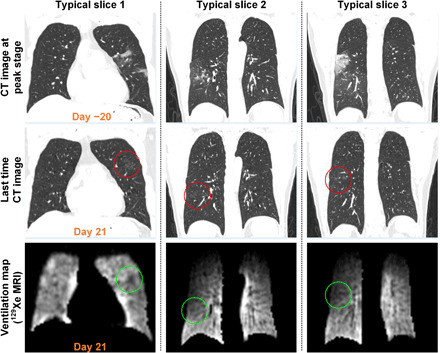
Lung images for subject 2. Serial chest CT images (top row: day −20, three slices; second row: day 21, three slices) and corresponding HP ^129^Xe gas MRI pulmonary ventilation maps (day 21) for one patient who underwent the final CT and gas MRI scans on the same day. In the final follow-up CT images, light GGOs are indicated by red circles. The same GGOs are indicated in the corresponding HP ^129^Xe gas MR images by green circles.
